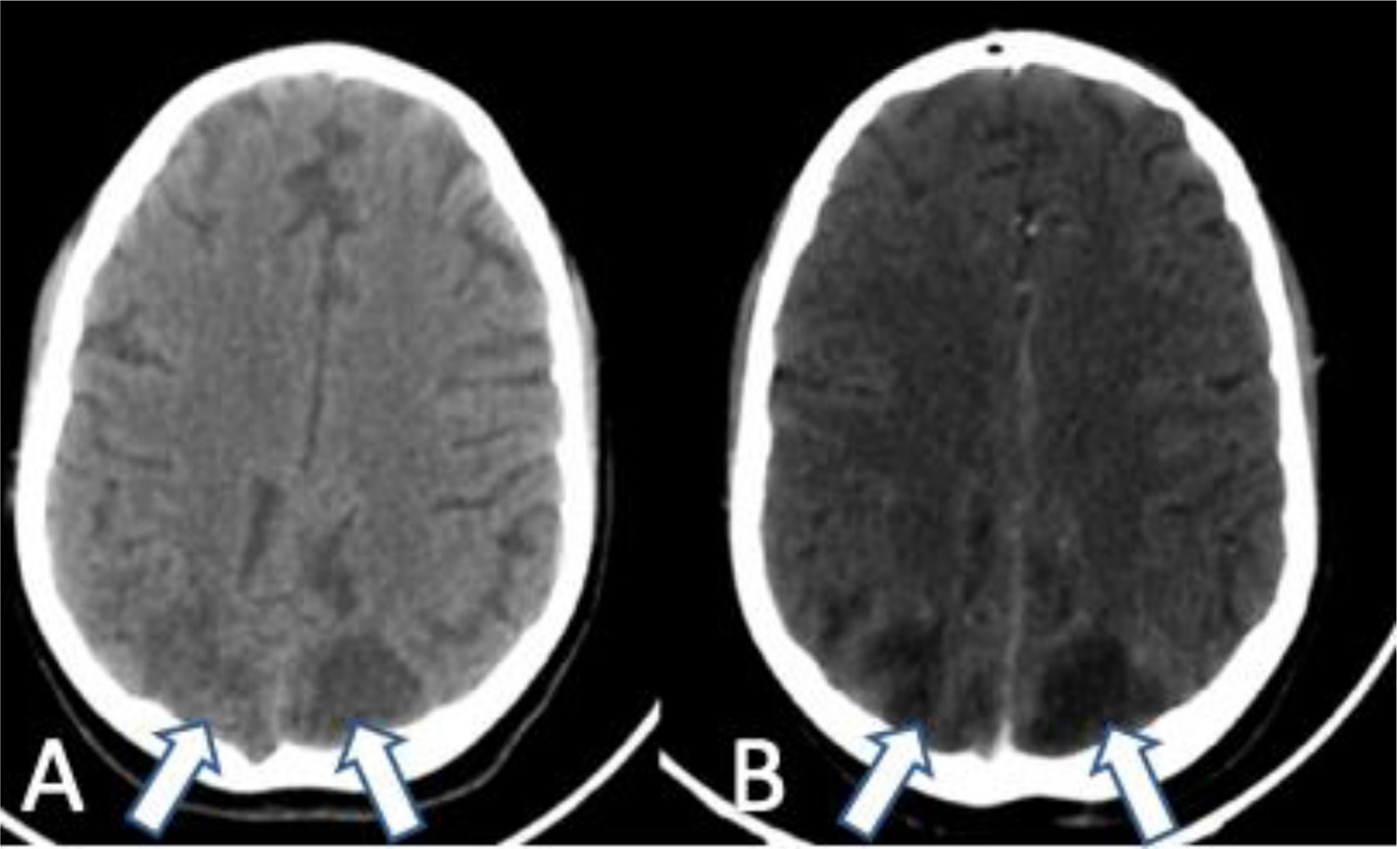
Axial CT obtained day 1 of admission, showing subtle bilateral, grossly symmetrical, cortical and sub-cortical posterior parietal hypodensities (arrows), unchanged before (A) and after contrast (B), consistent with PRES.
